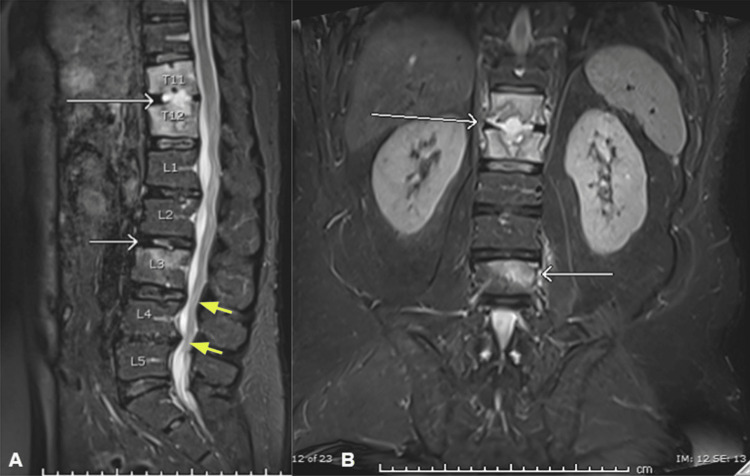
MRI at presentation showing inflammatory and degenerative changes (A) Sagittal MRI showing inflammatory process at T11-T12 and L2-3 disc levels (white arrows). Note the degenerative changes occurring in the lumbar spine, indicative of a spondylodegenerative condition (yellow arrows). (B) Coronal MRI showing the inflammatory process occurring in T11-T12 and L2-L3 disc levels (white arrows).
